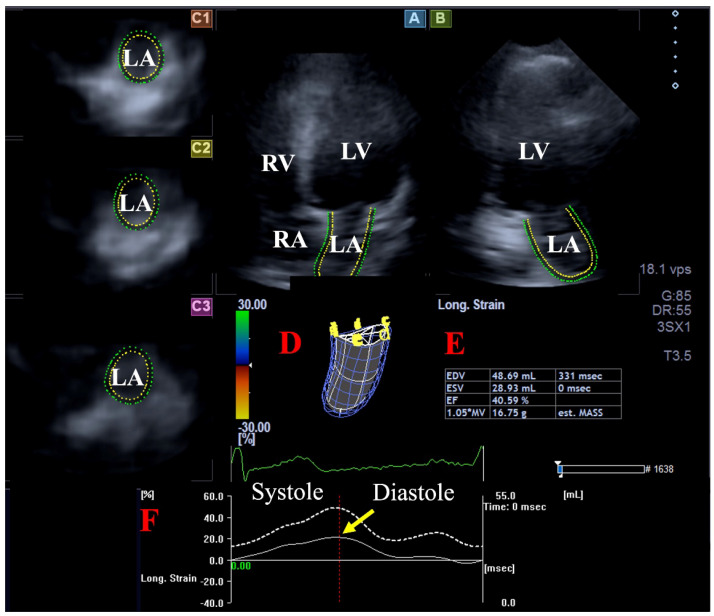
A three-dimensional (3D) speckle-tracking echocardiography-derived evaluation of global left atrial (LA) peak reservoir longitudinal strain (GLS) is presented: (A) apical four-chamber view, (B) apical two-chamber view and (C1) basal, (C2) midatrial, and (C3) superior LA short-axis views. A virtual 3D LA cast (D), LA volumes respecting the cardiac cycle (E) and time–LA volume change (dashed white line) and time–LA-GLS curves during the heart cycle (F) are also presented. On the twin-peak LA strain curve, the first peak (yellow arrow) represents the systolic reservoir phase of LA function (peak LA-GLS). Abbreviations: RV = right ventricle, RA = right atrium, LV = left ventricle, LA = left atrium, EDV = end-diastolic volume, ESV = end-systolic volume, EF = ejection fraction, and Long. = longitudinal.
